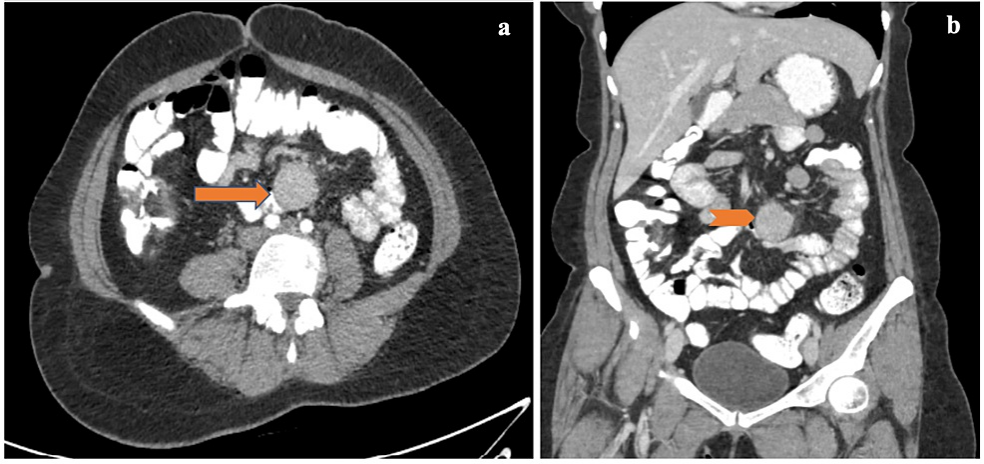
(a) Axial image of contrast-enhanced computed tomography showing homogenously enhanced lesion (solid arrow) in small bowel mesentery. (b) Coronal imaging showing homogenous lesion (solid chevron) at distal ileal mesentery.
